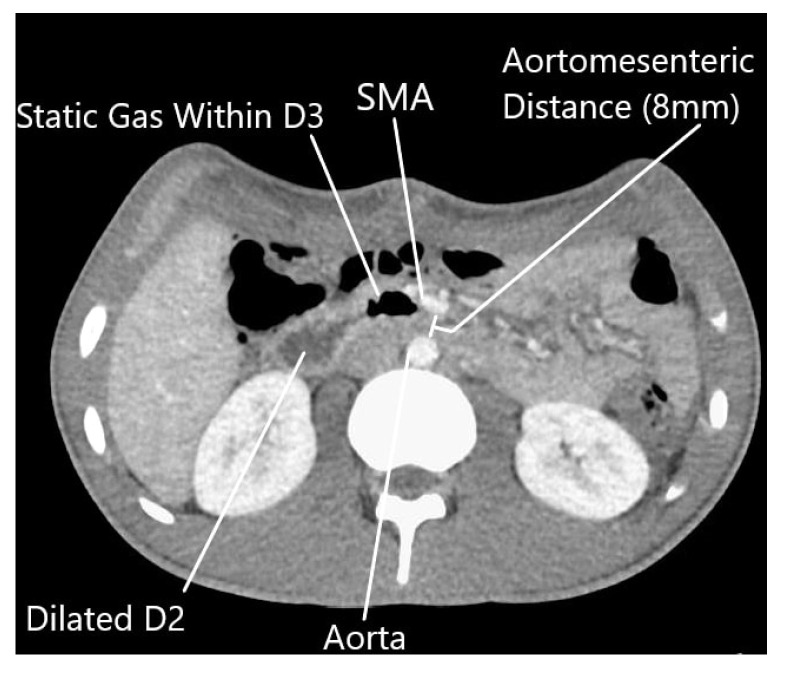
Abdominal computed tomography scan showing post-operative return of superior mesenteric artery to normal anatomic position, with reduced aortomesenteric distance (8 mm). (SMA—superior mesenteric artery, D2—second portion of the duodenum, D3—third portion of the duodenum).
